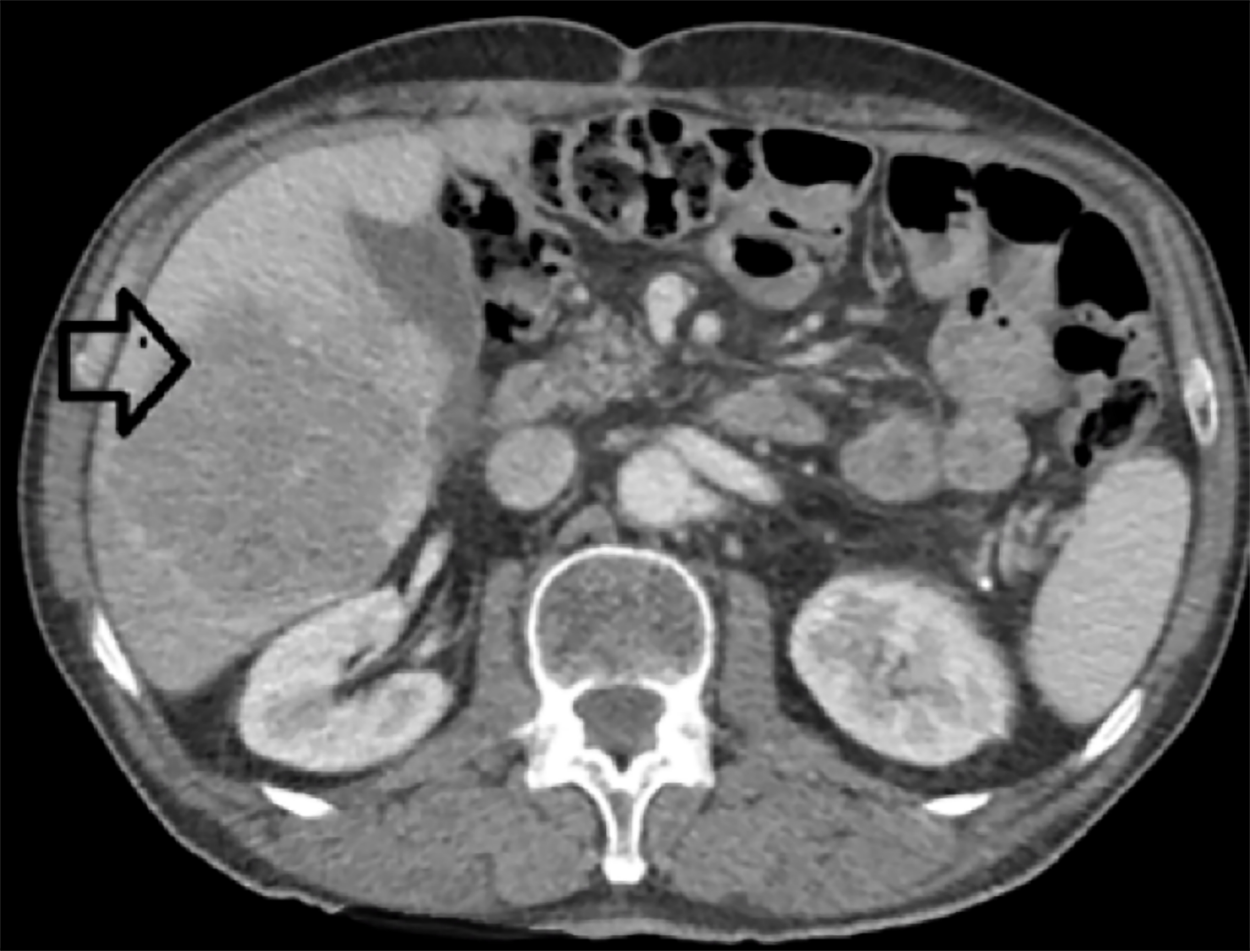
Computed Tomography Image Revealing a Single Liver Metastasis, Located in Segments 5 and 6 of the Liver
